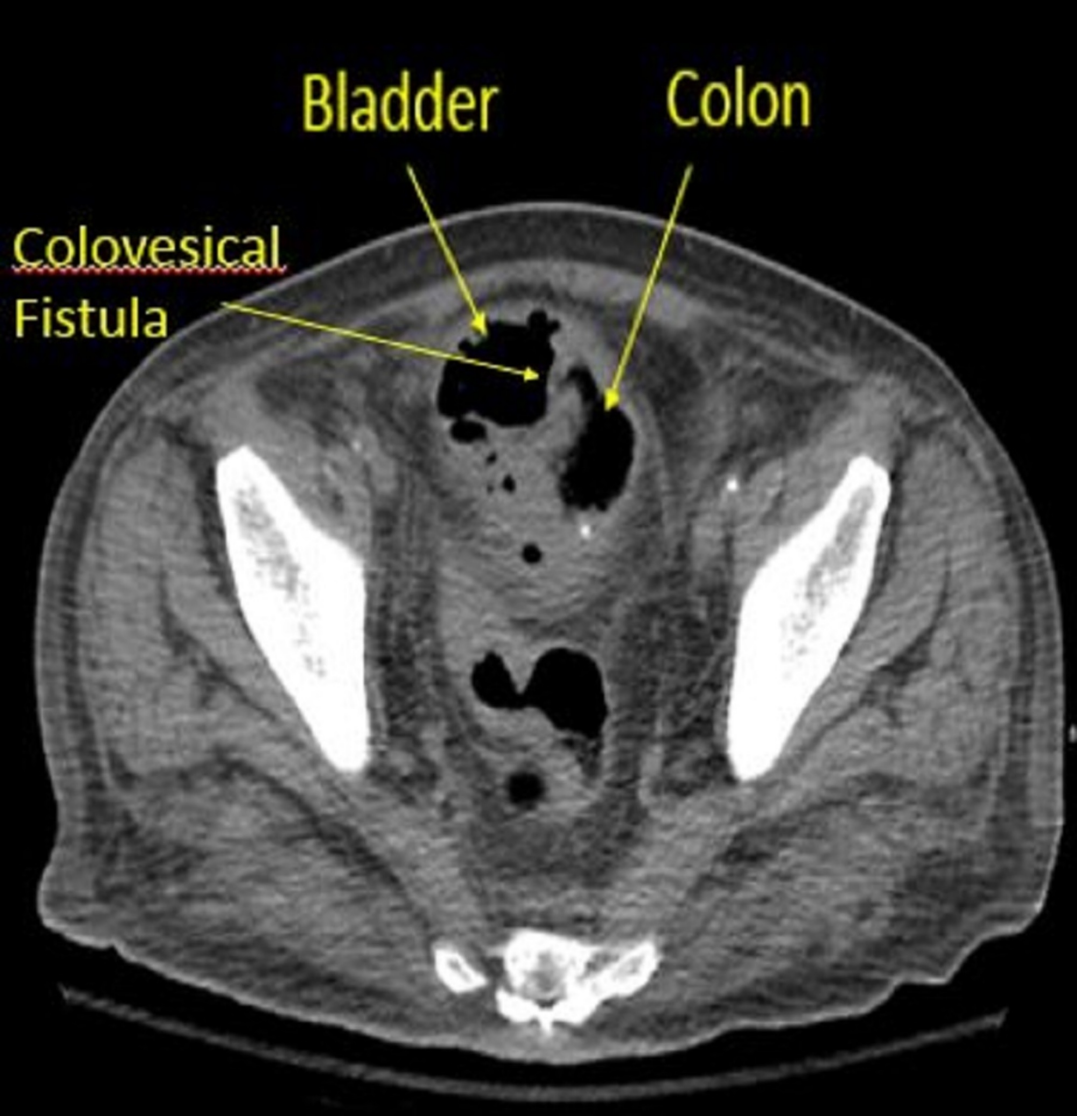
Computed tomography abdomen and pelvis scan showing colovesical fistula forming between colon and bladder with air in the urinary bladder.
